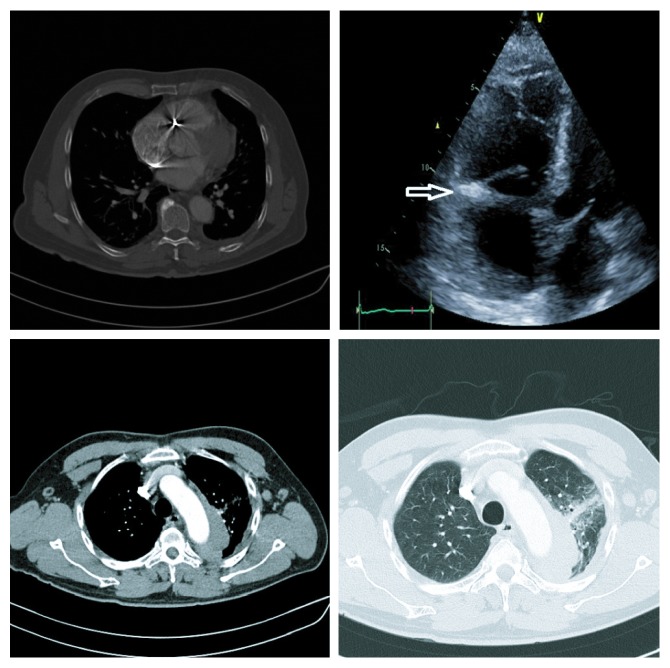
Thoracic CT shows hematoma around the aorta with no wall wound. Echocardiogram shows bullet in the tricuspid valve ring without perforation (arrow).
